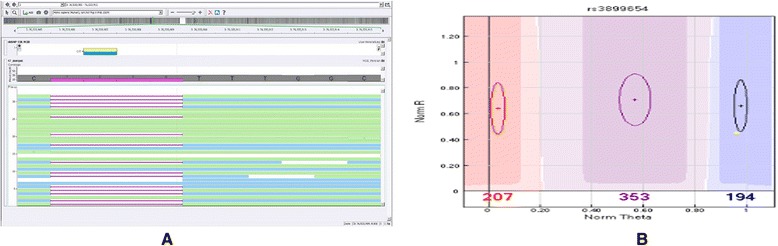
Impact of novel SNPs and indels in the vicinity of SNPs typed on bead chip on genotype calling. (A) Considered is an exemplary SNP of rs3899654, which has a novel heterozygous deletion of 2 bps upstream of the variant in KGP1 genome, that has CT call. (B) The typed marker in bead chip leads to inconsistent genotype call of CC.
